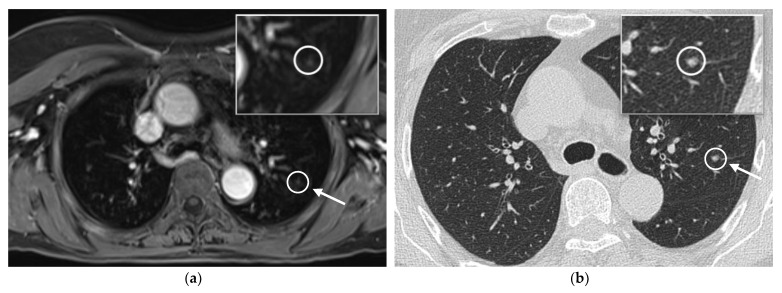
Example of the assessment of the lung in the 20-min-WB-MRI-protocol and the recommended further CT-scan: example of axial post-contrast T1w VIBE Dixon of the thorax (a) and CT-scan one week later (b) in a 73-year old patient with malignant melanoma and currently no evidence of disease. In the WB-MRI scan, a new pulmonary nodule (arrow) was detected and a further CT scan was recommended. Please note that the images have been cropped, as the skin tissue was part of the acquisition.
